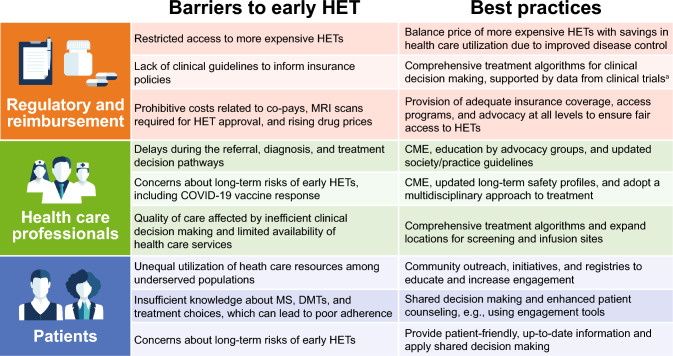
Proposed best practices for overcoming barriers to early high-efficacy therapy (HET) treatment in multiple sclerosis (MS) from the perspective of regulatory and reimbursement agencies, health care professionals, and patients. ^a^Anticipated clinical trial data from DELIVER-MS and TREAT-MS to support the benefit of early HET treatment in MS outcomes. *CME* continuing medical education, *COVID-19* coronavirus disease 2019, *DMT* disease-modifying therapy, *MRI* magnetic resonance imaging
